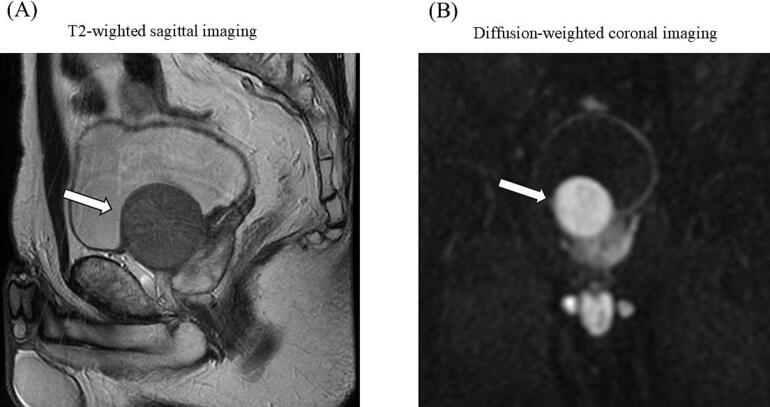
Pelvic MRI showing a well-circumscribed, homogenous tumor (arrows) with low-intensity on the T2-weighted image (A) and high-intensity on the diffusion-weighted image (B) arising from the anterior border of the prostate and compresses the bladder wall. MRI, magnetic resonance imaging.
